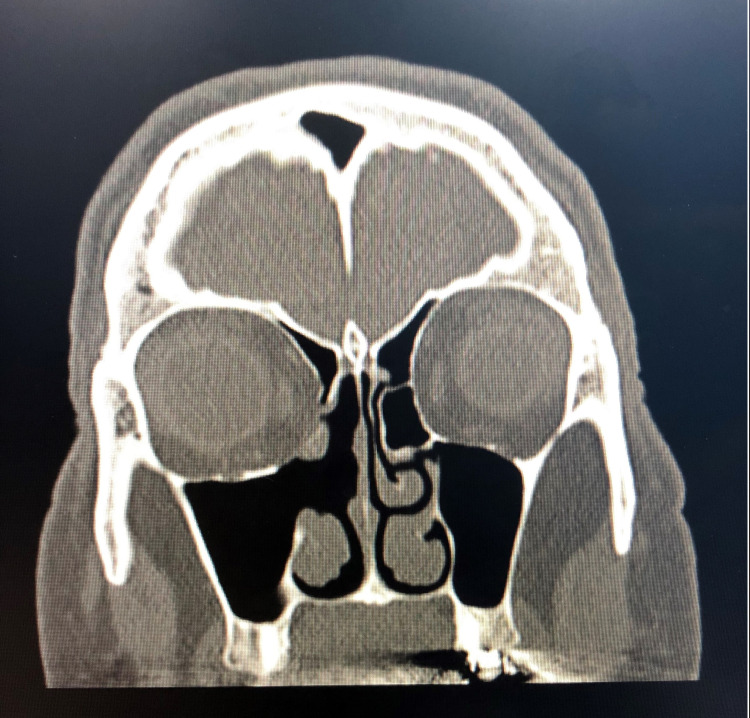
Postoperative coronal CT scan showing complete resolution of the lesion
